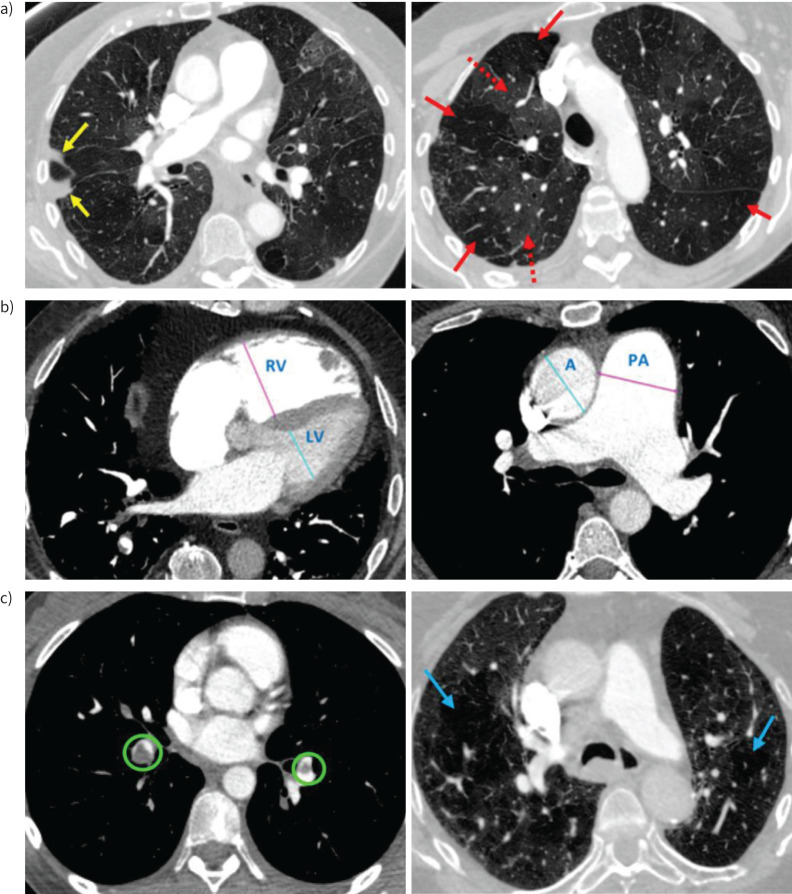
a) Examples of lung parenchymal features of chronic thromboembolic pulmonary hypertension (CTEPH) on computed tomography pulmonary angiography (CTPA). Axial CTPA images with standard lung windows from two different patients. The patient on the left showed lung infarction within the right middle lobe (yellow arrows). This typically appears as focal wedge-shaped consolidation at the lung periphery which resolves over time. Both patients exhibit mosaic perfusion. This refers to regional hyperattenuation (dashed red arrows) and hypoattenuation (solid red arrows) of the lung parenchyma, of which abnormal lung perfusion is a cause. It is important to note that neither lung infarction nor mosaic perfusion are specific to CTEPH and there is a broad differential diagnosis for both of these imaging findings. b) Examples of cardiac changes commonly seen in CTEPH on CTPA. Two axial CTPA images with standard soft tissue windows are shown. The right image shows marked dilatation of the right ventricle (RV) relative to the left ventricle (LV), with an RV/LV ratio of > 1.0, consistent with RV dysfunction. The left image shows dilatation of the pulmonary artery (PA) trunk relative to the ascending aorta (AA), with a PA/AA ratio of >1.0. c) Examples of CTEPH within left and right segmental disease (green circles), and emphysema lung disease in both lungs (blue arrows).
